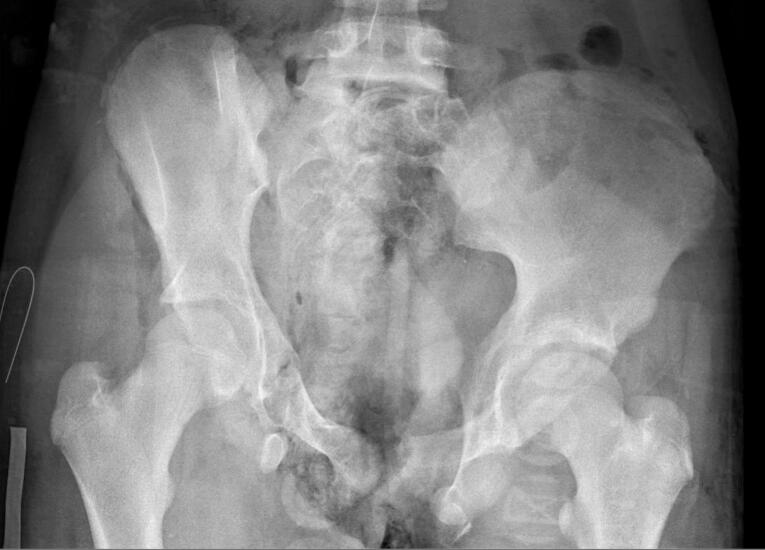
Anteroposterior Pelvic radiograph of 12 year old female with a Tile type C2 Pelvic fracture.
